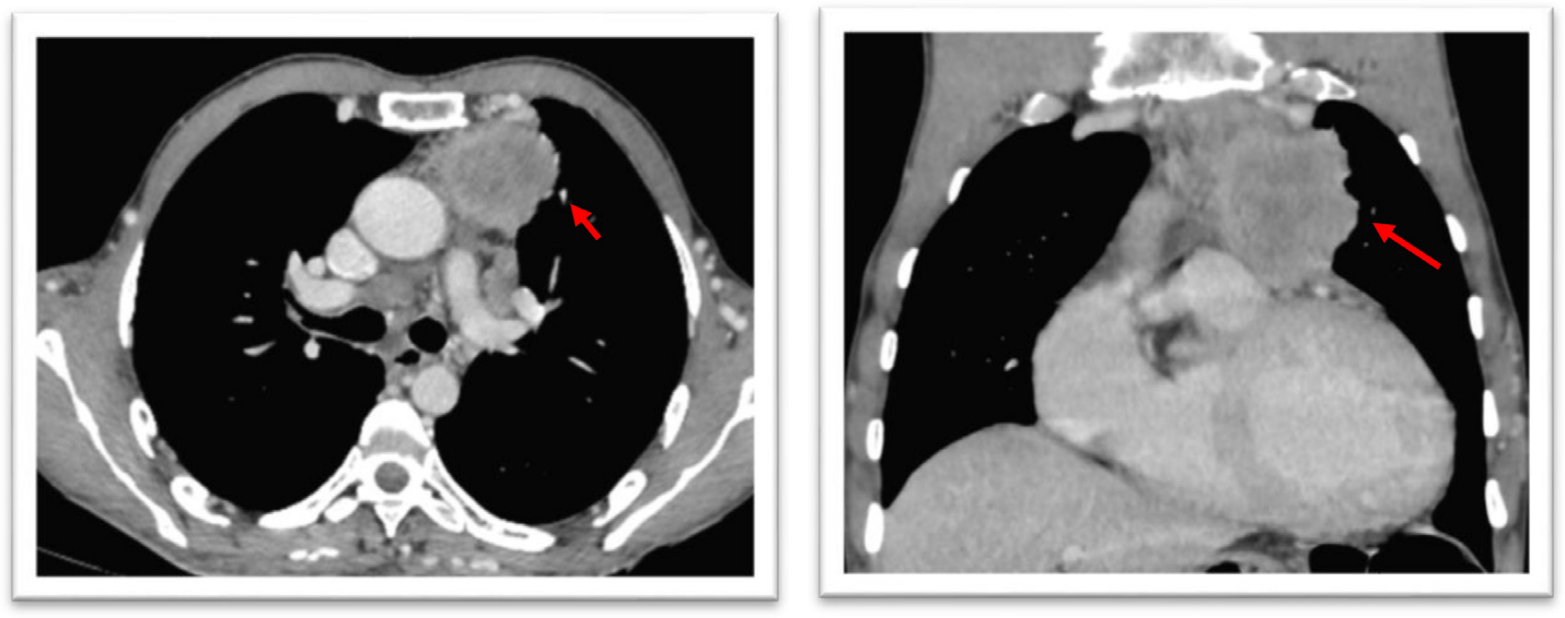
Axial-enhanced thoracic CT scan (A) and coronal reconstruction (B) showing: Locally advanced thymic carcinoma stage III of Masoaka system, well limited with poly-lobed contours, heterogeneously enhanced after contrast, containing intratumor necrosis. • Reaching aortic arch superiorly and on the trunk of the pulmonary artery and part of the right ventricle inferiorly, which are permeable and of normal caliber, with loss of the fatty separation border. • Externally: It invades the left mediastinal pleura and bulges toward the left upper lobe (red arrow).
